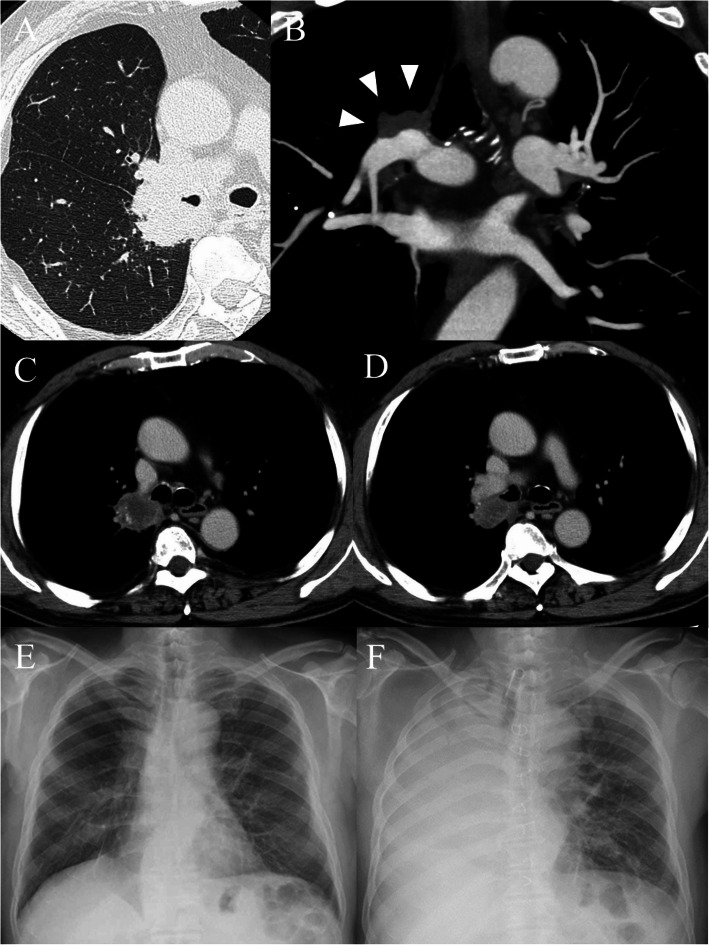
Chest computed tomography showing a tumor expanding over the remaining two lobes (a), compressing the pulmonary artery at the cut end of the superior trunk (b, arrowhead), adjoining to the azygos vein stump branching from the superior vena cava (b), and adhering to the membranous side of the right main bronchus (d). Compared with preoperative chest X-P (e), radiolucency was declining in the right upper lung field after pneumonia (f)
